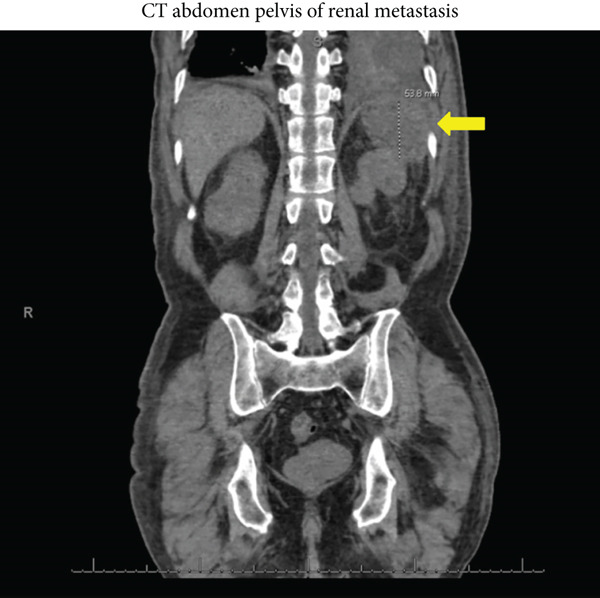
A CT abdomen pelvis in the sagittal view showing the patient’s left‐sided renal metastasis (yellow arrow) abutting the diaphragm.
